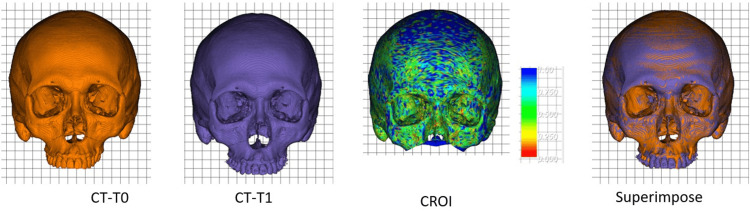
CROI registration The CROI was defined as the entire cranium, including the orbit. CT-T1 was superimposed on CT-T0 with reference to the CROI. CROI: cranial region of interest, CT-T0: 3DCT model at the initial visit, CT-T1: 3DCT model before orthognathic surgery, 3DCT: three-dimensional computed tomographic
